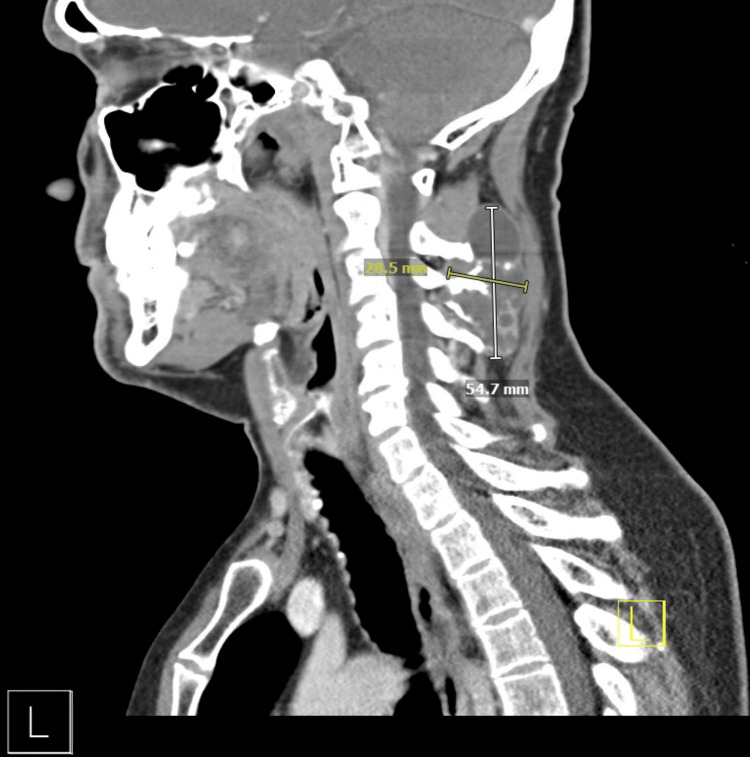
Contrast-enhanced CT of the neck. Sagittal image demonstrating an expansile multilocular cystic lesion centered within the C3 spinous process, measuring 28.5 mm x 54.7 mm.
